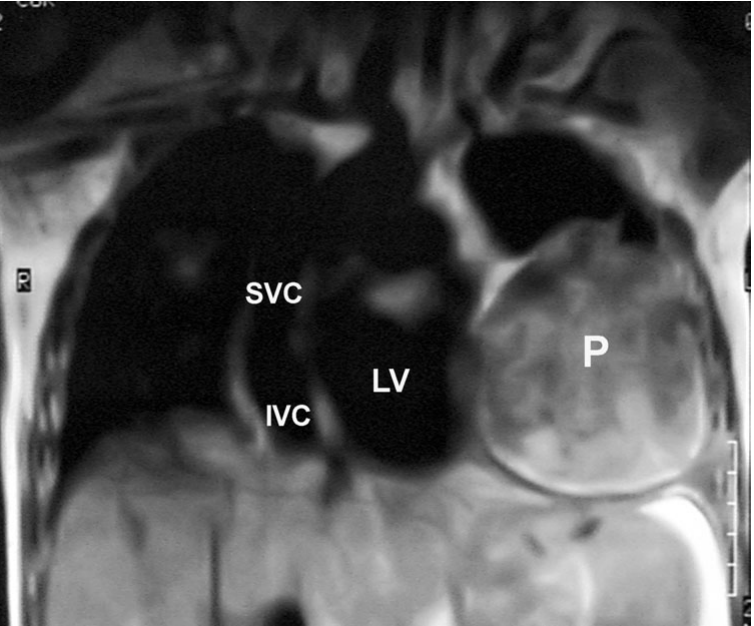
The MRI indicated a mass with inhomogeneous signals implying the presence of blood and clots in addition to calcification. (SVC = Superior Vena Cava; IVC = Inferior Vena Cava; LV = Left Ventricle; P = Pseudoaneurysm)
